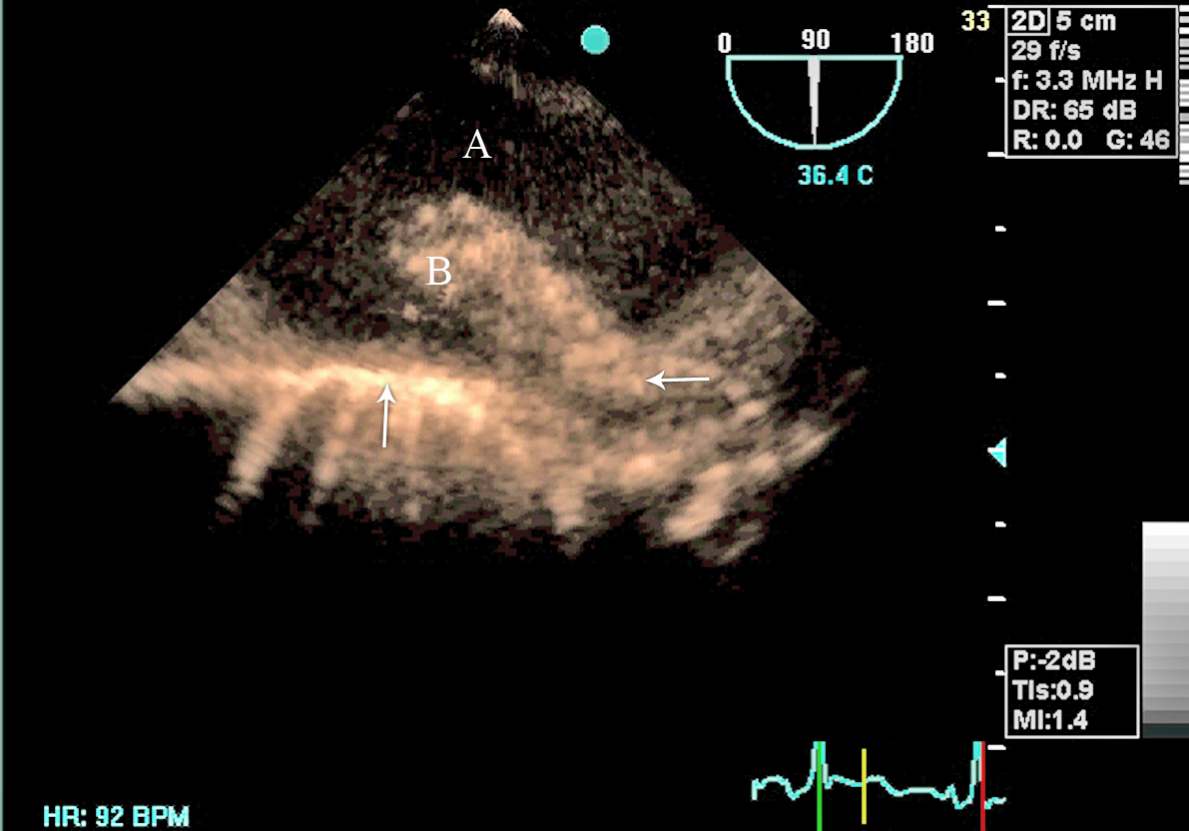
Transesophageal echocardiography (TEE) reveals a large mobile mass in the aortic arch. TEE is obtained at 90° angle and demonstrates the aorta and the aortic mass in the longitudinal view. Letter A shows the internal lumen of the aorta. Letter B stands for the atherosclerotic plaque. Horizontal arrow shows the attachment site of the atherosclerotic plaque to the aorta. Vertical arrow shows the normal adjacent aortic wall.
